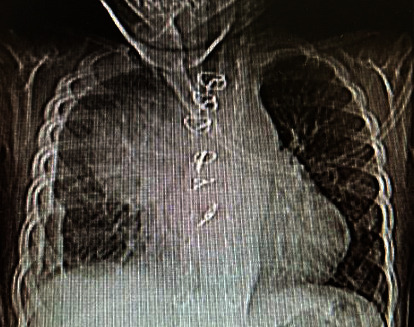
Chest X-ray in AP view showed a large mass in the right superior border.
